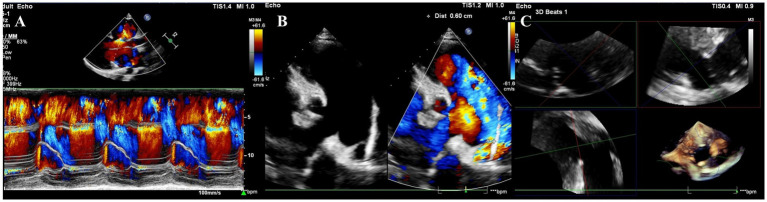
Pre-operative transthoracic echocardiography report. Detailed chamber sizes, VSD dimensions and Doppler gradients, valve regurgitation velocities, ductal flow, PASP estimation, RV functional indices (TAPSE, RVFAC), and pericardial / IVC findings are reported in Section 2.5.1 of the main text. (A) Apical four-chamber view with chamber enlargement and VSD; (B) VSD colour Doppler with bidirectional flow and tricuspid regurgitation; (C) Ductal colour/spectral Doppler for PASP estimation.
